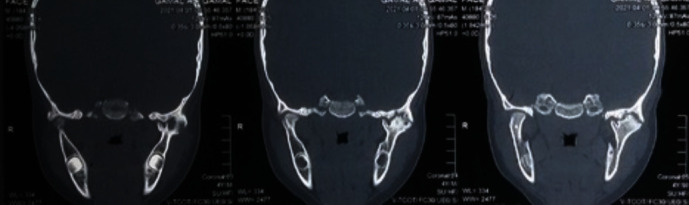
A coronal CT scan view showing left TMJ ankylosis.
